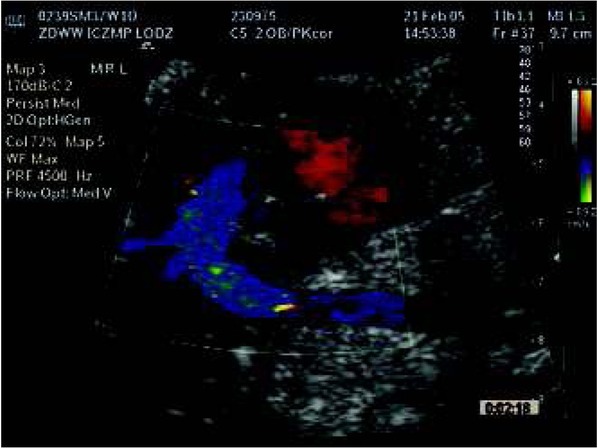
Fetal aortic arch in long axis scan with color Doppler showing normal laminar blood flow. Ryc. 5. Obraz łuku aorty płodu w osi długiej w badaniu echokardiograficznym z kolorowym Dopplerem pokazujący lamilarny kierunek przepływu krwi.
